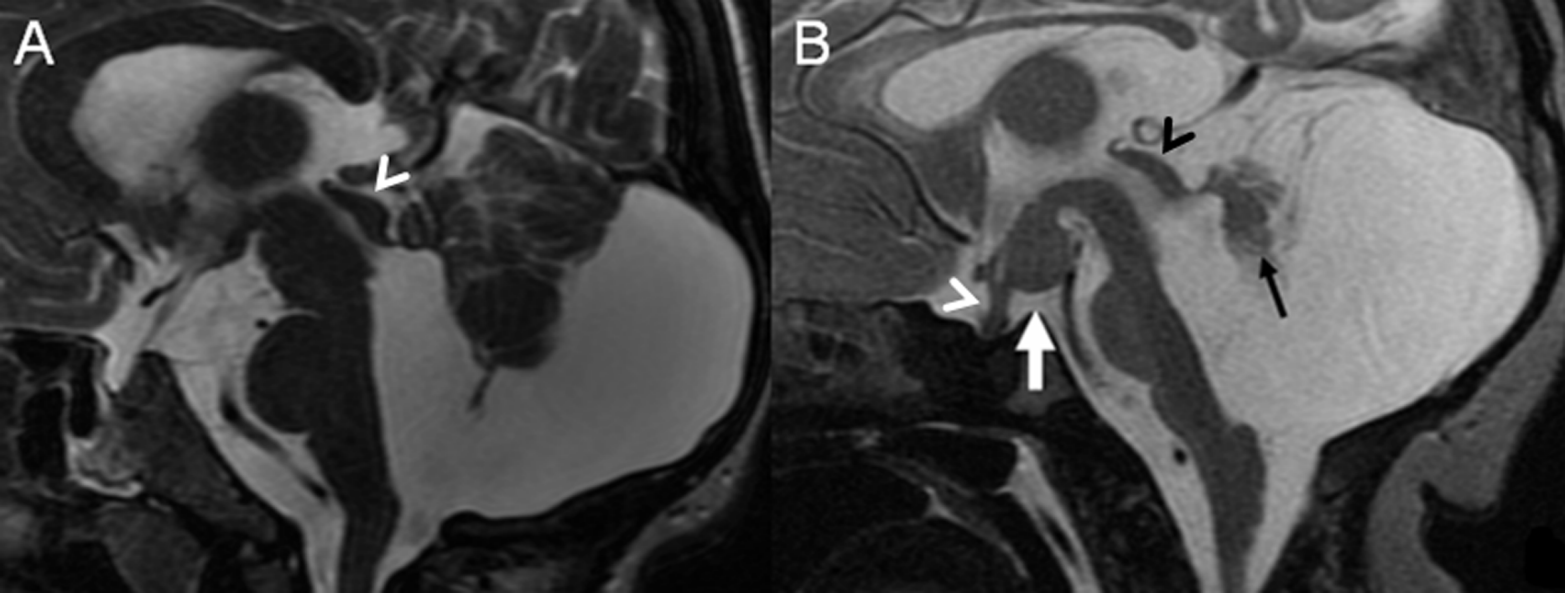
Midsagittal T2-weighted MR images of a 22-year-old woman (A) and a 2-day-old neonate (B), modified from Poretti A et al, AJNR, 2008, with permission) with OFD VI reveal an enlarged posterior fossa with marked retrocerebellar CSF collection. Additionally, in both patients the brainstem appears dysmorphic: in A the midbrain is thickened, the tectum dysplastic (white arrow head), and the pons short; in B there is elongation of the mesencephalon, reduced size of the pons, and dysplasia of the tectum (black arrow head). In both patients the cerebellar vermis is hypoplastic and its remnants are dysplastic (black arrow in B), the massa intermedia is prominent, and in B a hypothalamic hamartoma is seen (white arrow) and the pituitary stalk appears thickened (white arrow head).
